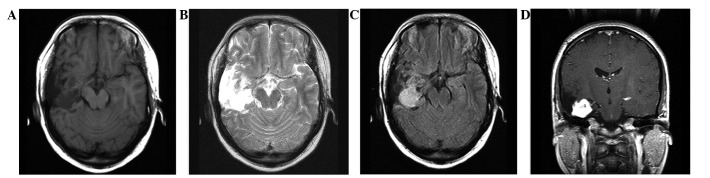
MRI of the recurrent tumor. (A) T1WI indicating tumor recurrence *in situ*, with a homogeneous and hypointense signal. (B) T2WI indicating a hyperintense lesion. (C) No obvious peripheral edema on FLAIR sequence imaging. (D) Intense enhancement of the recurrent lesion, shown by contrast-enhanced imaging. MRI, magnetic resonance imaging; WI, weighted imaging; FLAIR, fluid attenuated inversion recovery.
